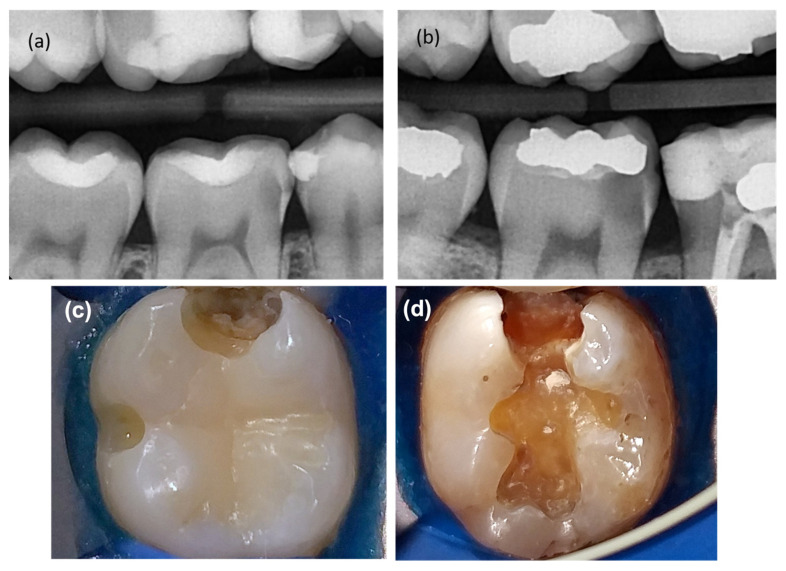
(a) Bitewing preoperative radiograph for first mandibular molar with deep carious lesion shows that the lesion involves the inner quarter of dentine with a radio-dense zone (hard or firm dentine) between caries and the pulp. (b) Bitewing preoperative radiograph for second mandibular molar with extremely deep carious lesion shows the lesion penetrating the entire thickness of the dentine with a radio-dense zone located within the pulp chamber indicative of tertiary dentine. (c) Intraoral image shows a rapidly progressing lesion and the yellow color of the demineralized dentine with soft and wet appearance of the surface texture. (d) Intraoral image shows a slowly progressing lesion and the brown color of the demineralized dentine with firm/dry appearance of the surface texture.
